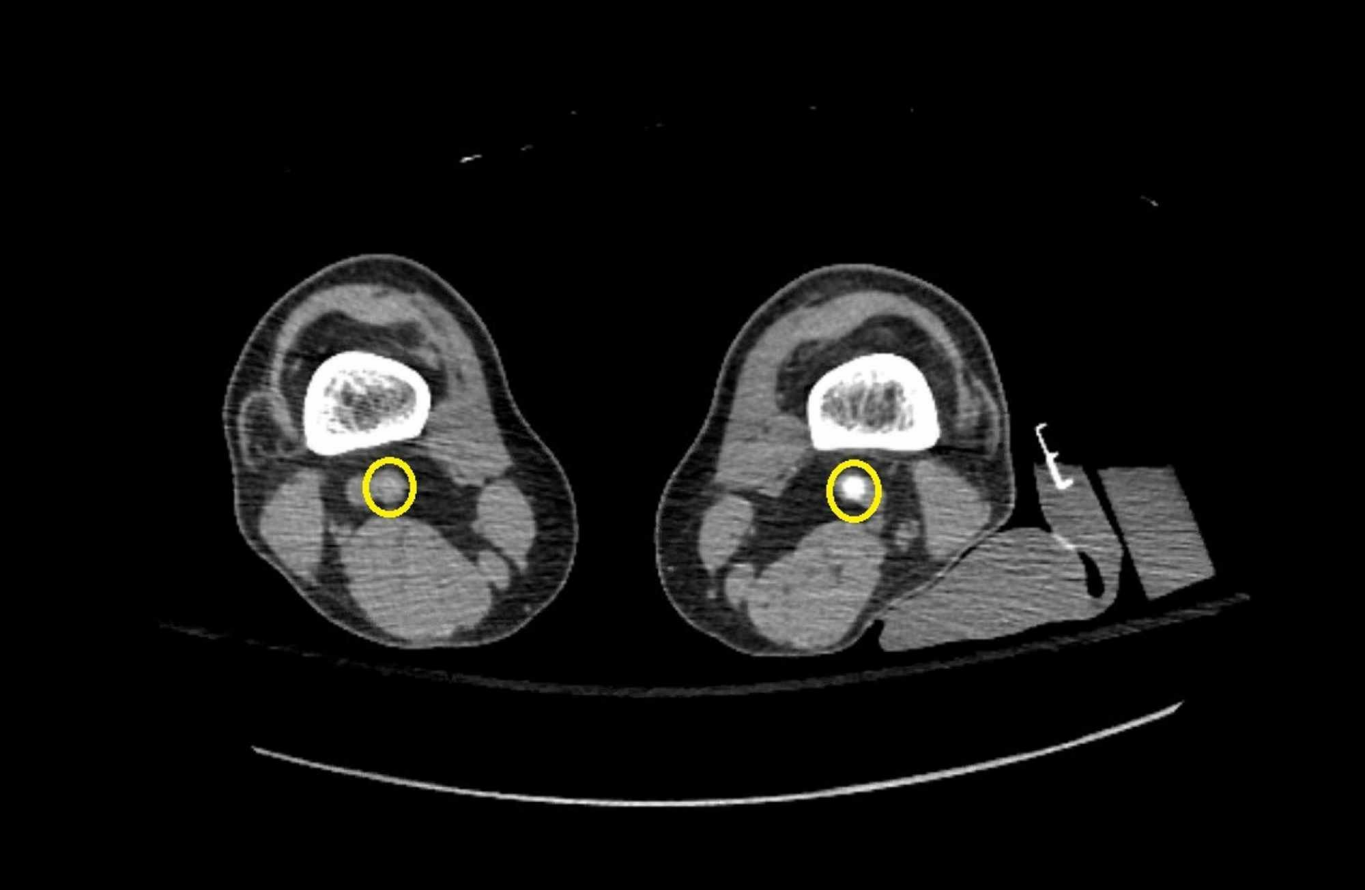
CT scan of the bilateral lower extremities with intravenous (IV) contrast Demonstrating acute occlusion of the right popliteal artery at the level of the distal femur/knee and patency of the left popliteal artery. Bilateral popliteal arteries are indicated by yellow circles.
